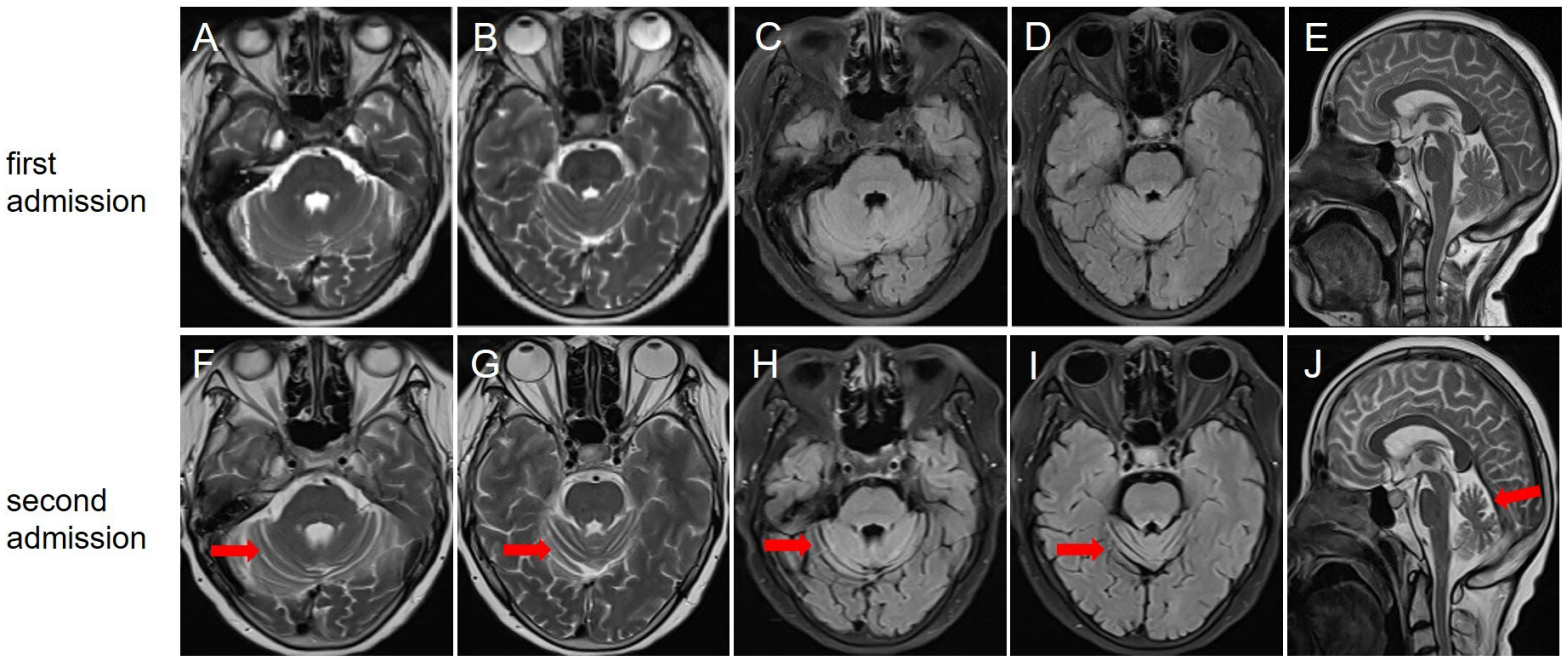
Brain magnetic resonance imaging at the first (A–E) and second (F–J) admission. T2-weighted (A, B), fluid-attenuated inversion-recovery (C, D), and sagittal view (E) showed no significant cerebellar atrophy; (F, G) T2-weighted showed that the bilateral cerebellar hemispherical sulci were significantly wider and deeper than before; (H, I) Fluid-attenuated inversion-recovery sequence showed cerebellar atrophy; (J) Sagittal view indicated cerebellar atrophy.
